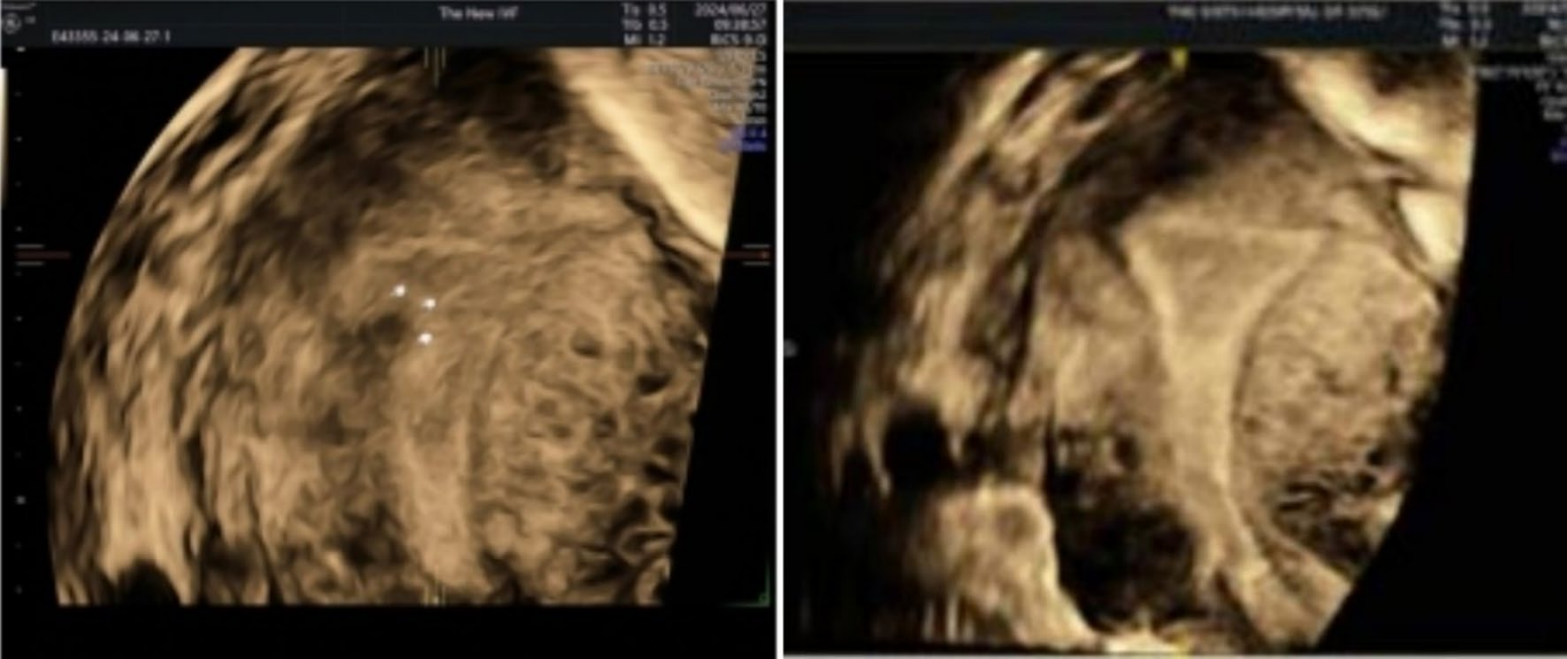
Three-dimensional ultrasound images of before and after cold instruments for hysteroscopic myomectomy
